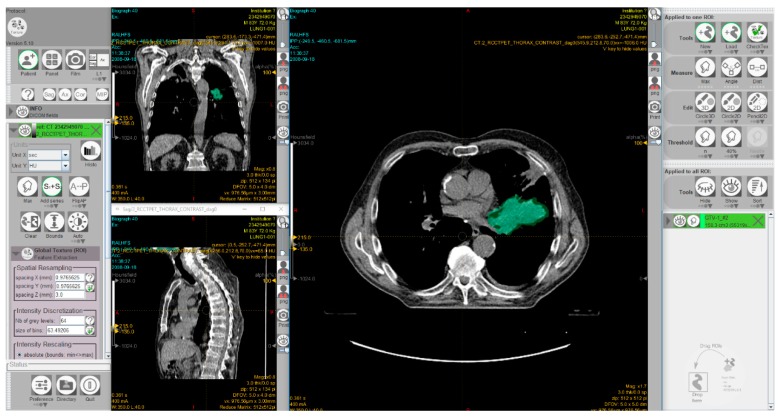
A screenshot of the graphical user interface of the freeware software LIFEx [70] for radiomic feature extraction. The interface shows computed tomography images of a patient with non-small cell lung cancer. The 3D volume of the gross tumor volume, manually delineated by a radiation oncologist, is shown in green. This exam was taken from the ‘NSCLC-Radiomics’ dataset [72,73] which is publicly accessible via ‘The Cancer Imaging Archive’ (TCIA; https://www.cancerimagingarchive.net).
